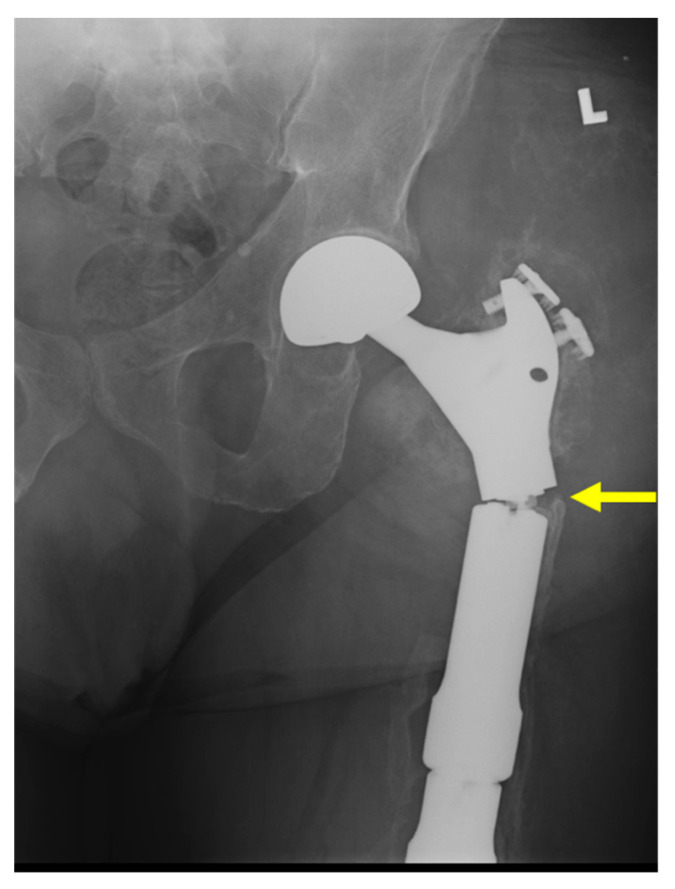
Plain radiograph of a 65-year-old male with history of femoral osteosarcoma treated with resection and endoprosthetic reconstruction, now presenting with frank prosthesis fracture (yellow arrow). Patient reported hearing a crack while standing and turning.
